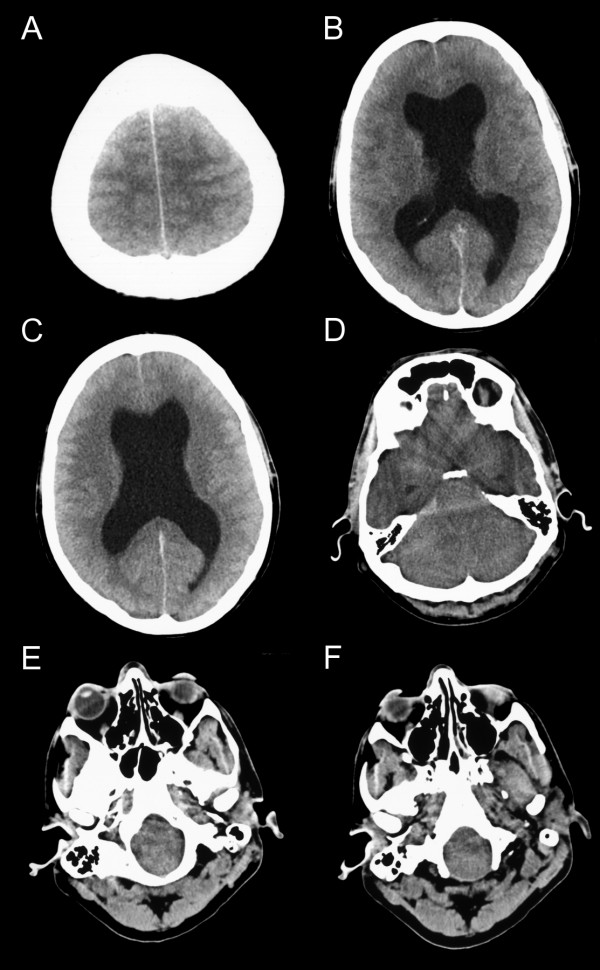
CT brain scan signs of hydrocephalus, high intracranial pressure and brain stem herniation. Brain CT (axial slices) in a male patient in his 30's who died of brain stem herniation after completing a marathon. The CT shows (A) loss of the rostral cerebral sulci suggesting increase in ICP, (B) and (C) a large hydrocephalus with widening of both temporal horns. The grey matter can still be differentiated from the white matter, but all sulci are lost. This suggests that the brain oedema is of relative recent onset and massive tissue ischaemia has not yet occurred. (D) Compression of the fourth ventricle with dilatation of the third ventricle and the caudal aspect of both temporal horns. This is observed with considerable brain oedema and obstructive hydrocephalus. (E) Herniation of the medulla and pons into the foramen magnum. (F) The tonsils are located at the level of the dens which is a good indicator for foramen magnum herniation. (All images are from the case presented here).
